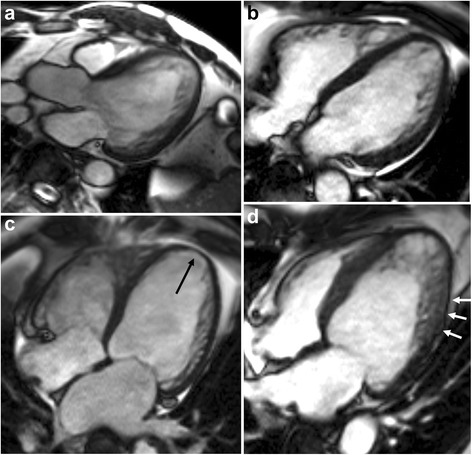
Types of LV non-compaction or hypertrabeculation on long-axis cine MR images. a Global type in a patient with isolated LVNC, b apical type in a patient with non-isolated LVNC, c) non-apical type in a patient with DCM, d) global type with severe wall thinning (arrows) in the compacted myocardium. Note absence of trabeculation in the apex (arrow) in the non-apical type (c)
